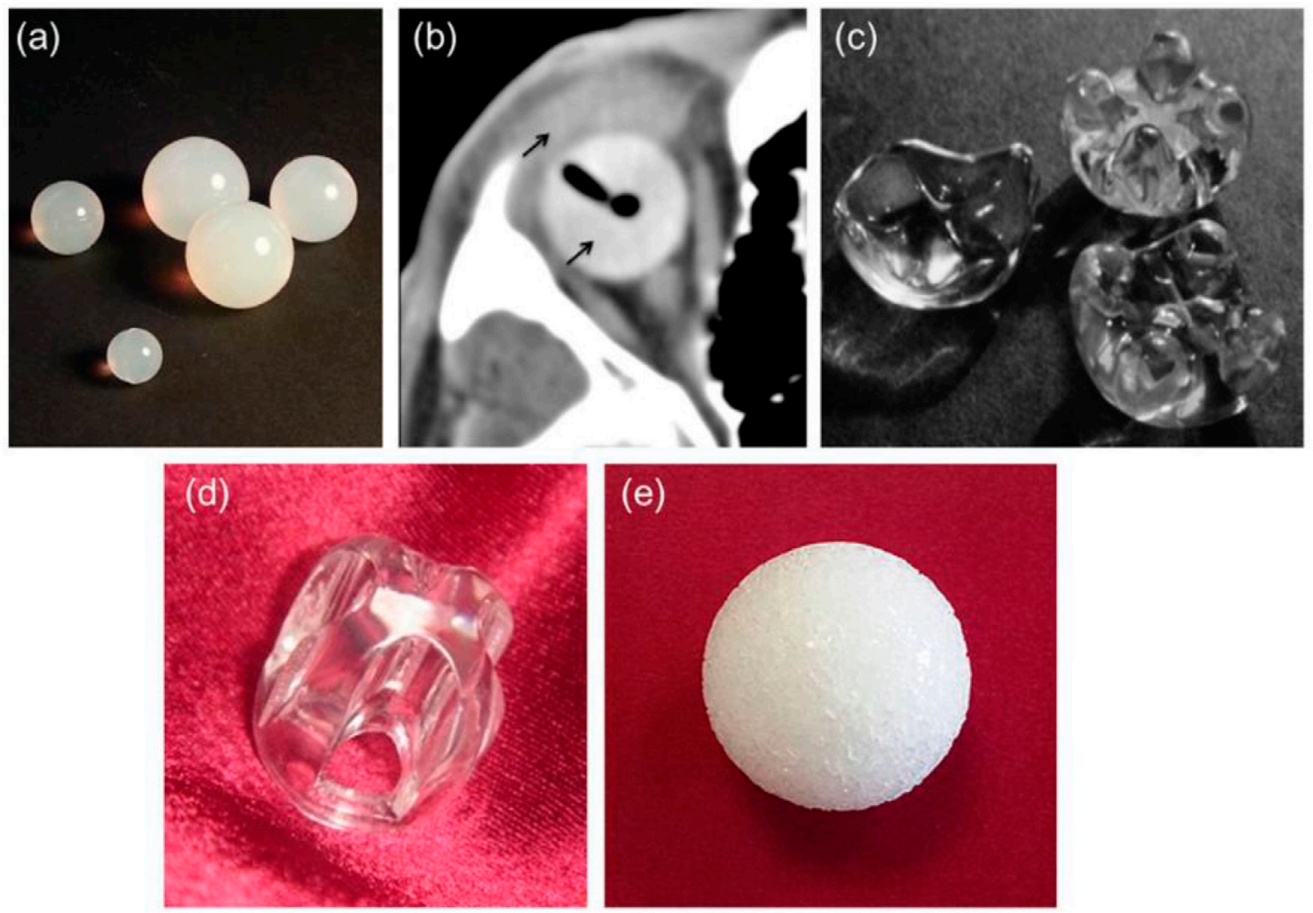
(A) silicone spheres, (B) axial CT image of an acrylic spherical implant with ocular prosthesis implanted *in vivo* (thin black arrows), (C) acrylic orbital implants of the “Allen family” (Iowa implant and its conformer—upper right corner and left, respectively; universal implant—lower right; the size of the implants around 20 mm 20 mm), (D) Castroviejo implant, and (E) porous sphere. Reproduced with permission from (Adams et al., 2014).
